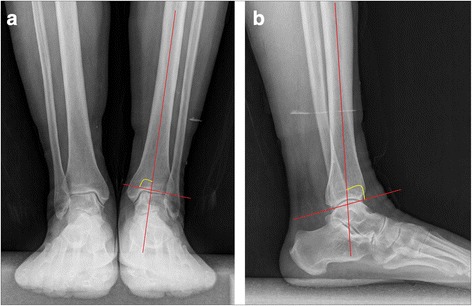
Radiographic measurements. a TAS angle. b TLS angle
